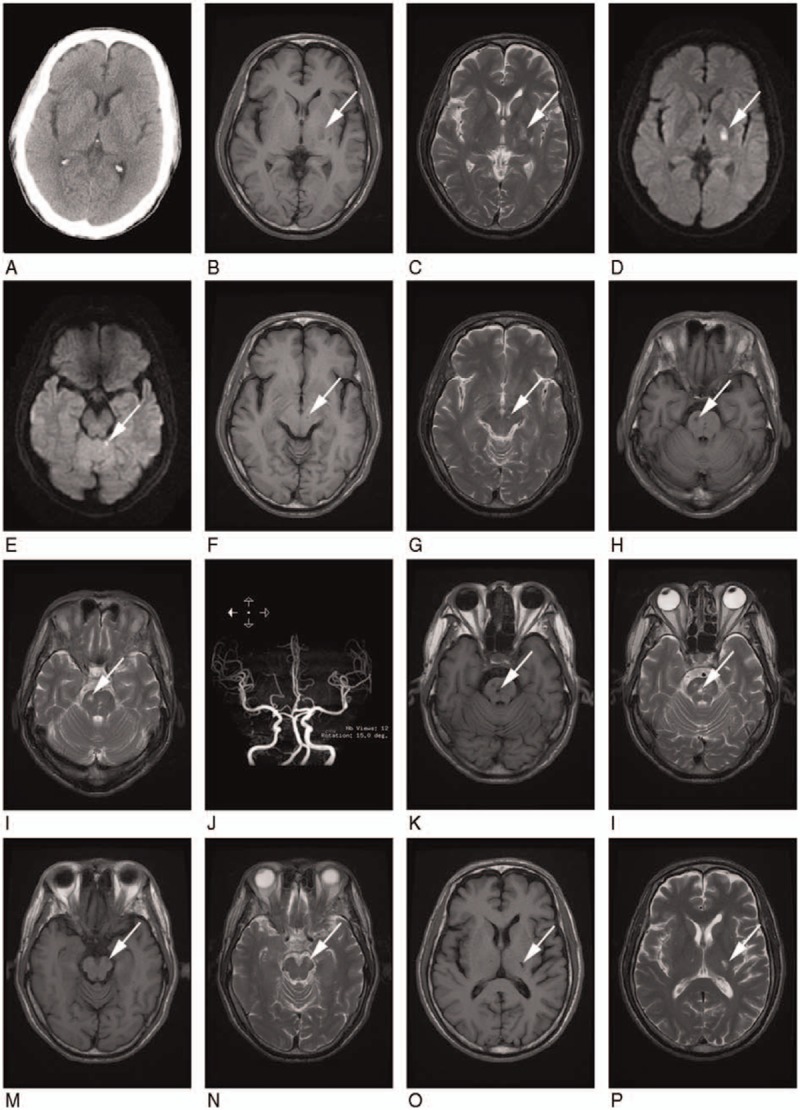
Brain imaging data. At the first admission, a brain CT scan was normal (A), but brain MRI and DWI showed new onset of lacunar infarction involving the left basal ganglia (B: T_1_ WI, C: T_2_ WI, D: DWI), the left vermis (E: DWI); and other lacunar infarction in the left side of the mesencephalon (F: T_1_ WI, G: T_2_ WI), and also the right side of the pons (H: T_1_ WI, I: T_2_ WI). MRA indicated no intracranial artery stenosis, but had stiff blood vessels (J). At the second admission, images revealed multiple lacunar infarcts observed in the pons varolii (K: T_1_ WI, L: T_2_ WI), the left side of the mesencephalon (M: T_1_ WI, N: T_2_ WI), and the right side of the thalamus (O: T_1_ WI, P: T_2_ WI). CT = computed tomography, DWI = diffusion weighted imaging, MRA = magnetic resonance angiography, MRI = magnetic resonance imaging.
